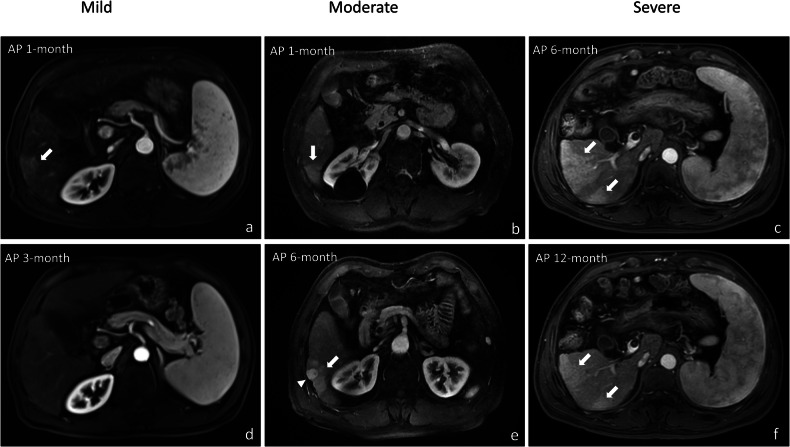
Severity of THID Following DEB-TACE. Mild THID (a, d): a axial T1-weighted image in the arterial phase shows abnormal parenchymal enhancement in Segment 6 (arrow) at 1 month post-DEB-TACE, which resolves by the 3-month follow-up (d). Moderate THID (b, e): b axial T1-weighted image in the arterial phase shows abnormal enhancement in Segment 6 (arrow) observed at 1 and 6 months post-DEB-TACE. e By 6 months, increased intensity of enhancement is noted surrounding a new metastatic lesion (arrowhead) in S6. Severe THID (c, f): c axial T1-weighted image in the arterial phase shows abnormal parenchymal enhancement involving Segments 5 and 6 (arrows) at 6 months post-DEB-TACE, with persistent but diminished enhancement evident at the 12-month follow-up (f)
